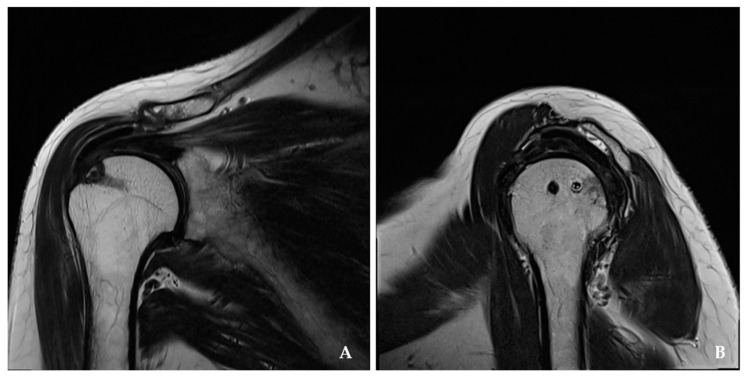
Augmented partial repair with the acellular dermal allograft was well preserved on-lay to the partially repaired rotator cuff tendons at the postoperative 1 year, and the medialized anchor position is notable. (A) magnetic resonance imaging of T1 coronal section. (B) magnetic resonance imaging of T1 sagittal section.
